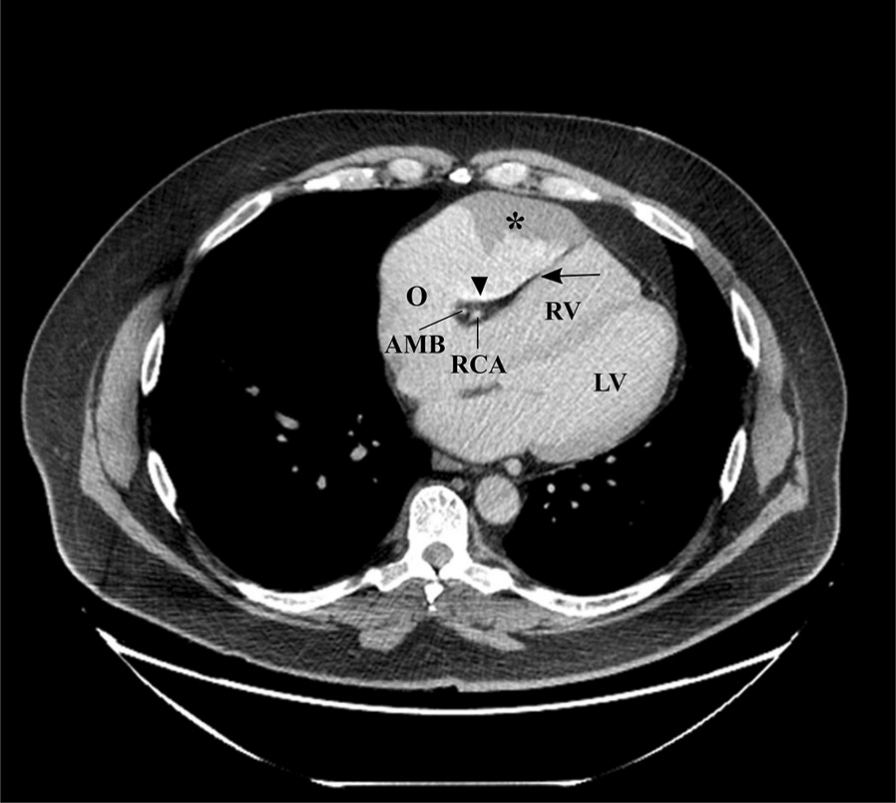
Computed tomography image showing the right atrial appendage aneurysm and an intraluminal filling defect. Axial computed tomography image (2.5 mm slice thickness) obtained after administration of intravenous iodinated contrast in soft tissue window centered at the level of the heart. There is aneurysmal dilatation of the right atrial appendage causing marked mass effect on the right ventricle, deforming its free wall (arrow). Note the 7.6 × 3 cm area of hypoenhancement/filling defect (asterisk) at the tip of the right atrial appendage, which could be the result of either slow flow with poor mixing of contrast or thrombus formation. Notice the layering of the iodinated contrast in the dependant portion of the right atrial appendage, more consistent with slow flow and poor mixing of contrast (arrowhead). The right coronary artery and acute marginal branch with surrounding epicardial fat are seen in the atrioventricular groove. A, RAA aneurysm; O, ostium of RAA; LV, left ventricle; RV, right ventricle; RCA, right coronary artery; AMB, acute marginal branch
